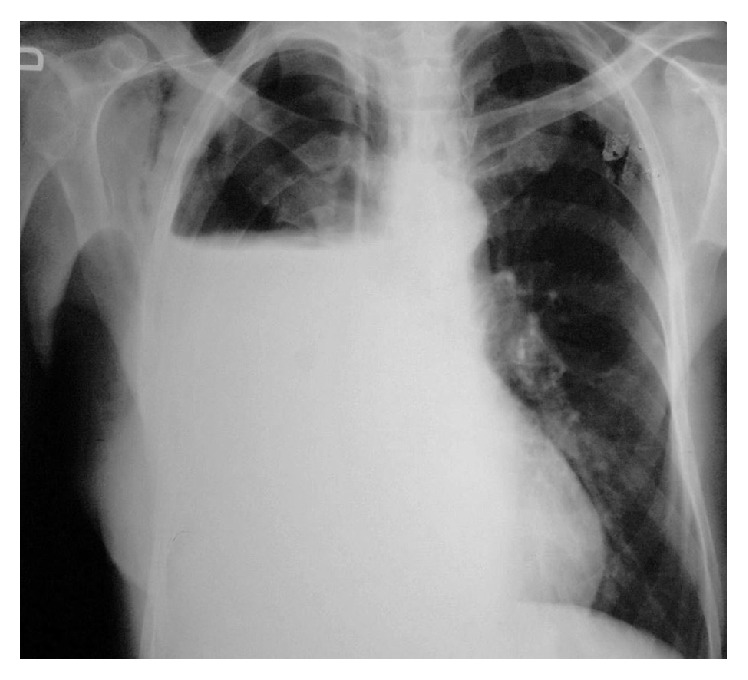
Chest X-ray shows hydroaeric level in the right hemithorax, suggesting state after pneumonectomy.
